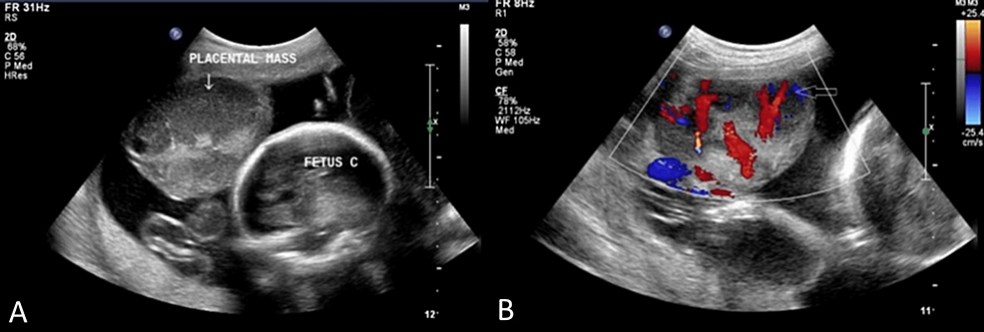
(A) An ultrasonographic image with a 5 MHz curvilinear probe shows a well-defined hypoechoic mass lesion (solid white arrow) arising from the edge of the placenta and protruding into the amniotic cavity. Three vessels in the umbilical cord are seen. (B) A color Doppler image with a 5 MHz curvilinear probe shows vascularity within the hypoechoic placental mass lesion (open white arrow).
